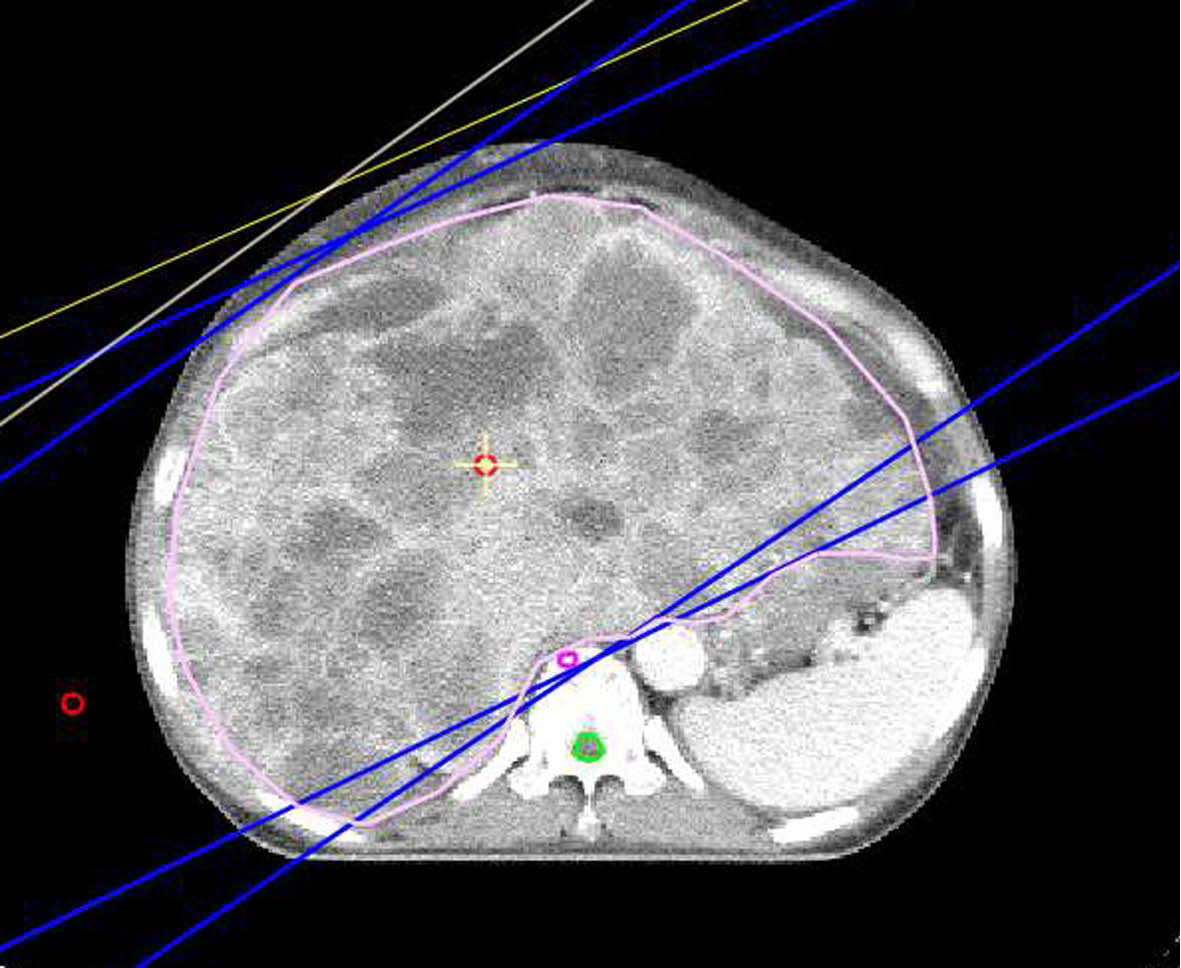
Central axial computed tomography slice showing the three-dimensional conformal radiotherapy plan. The treatment target was the whole-liver. Two radiation fields, with left anterior oblique and right posterior oblique beams, both consisting of 15 MV X-rays, were used to cover the target.
